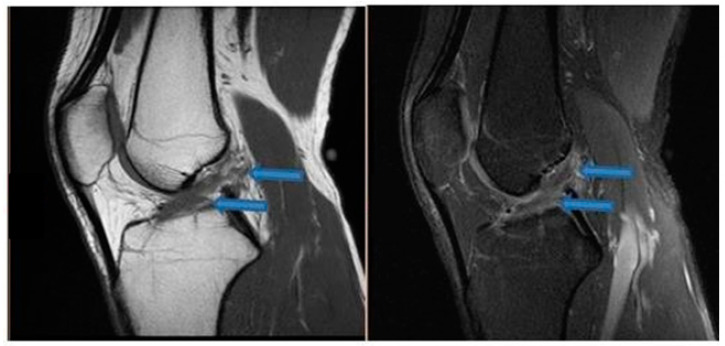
Sagittal T1- and T2-weighted MRI of the knee showing partial healing of the ACL indicated by blue arrows (9 November 2020).
